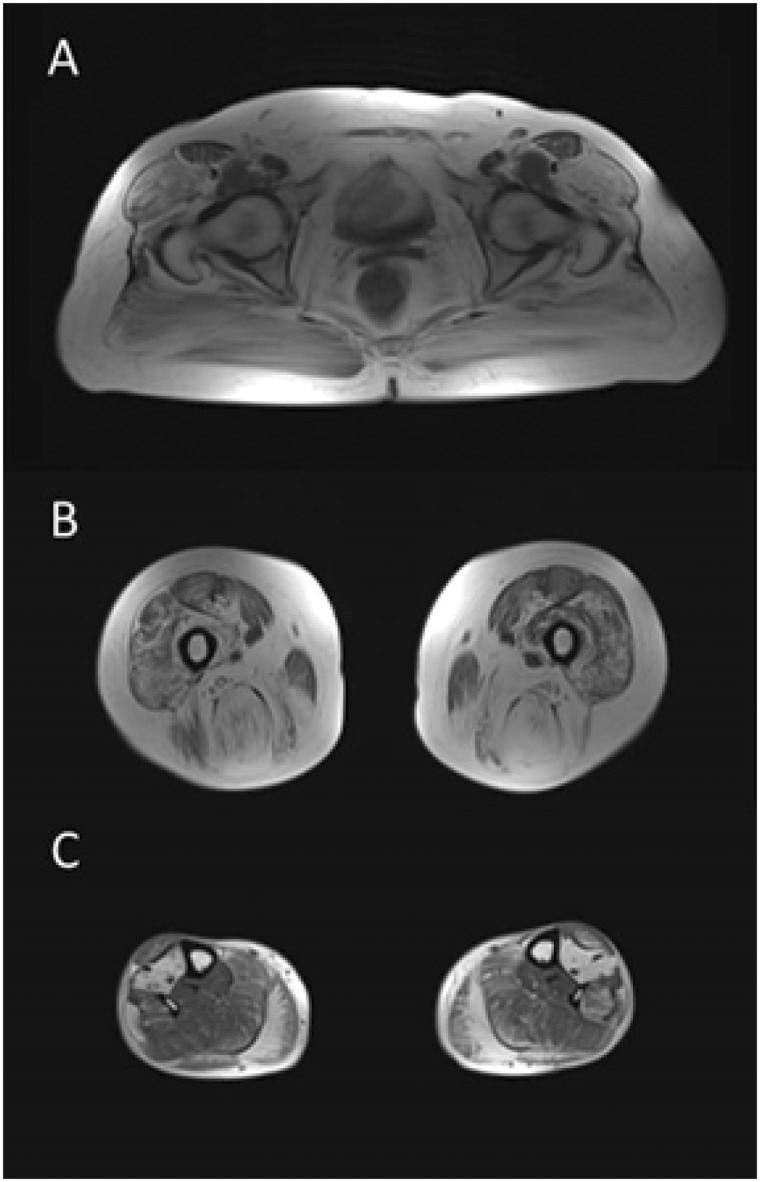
Muscle MRI from Case 2. The muscle MRI study (T_1_-weighted sequences) showed consistent abnormalities in gluteal (A), thigh (B) and calf (C) muscles, with confluent areas of increased signal or end-stage appearance but relative sparing of certain muscles, especially at the distal level, in keeping with the clinical and electrophysiological findings.
